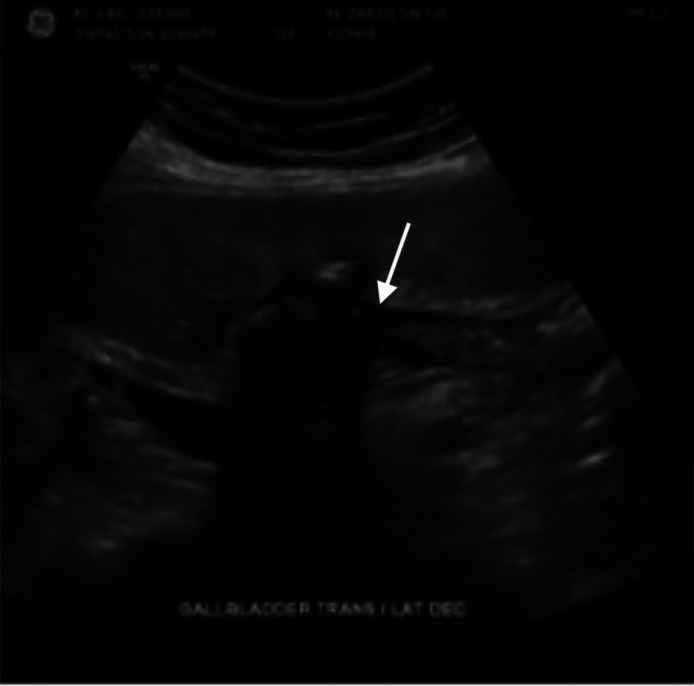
Grayscale ultrasound image. The image shows the choicysto-enteric fistula with the duodenum (white arrow).
